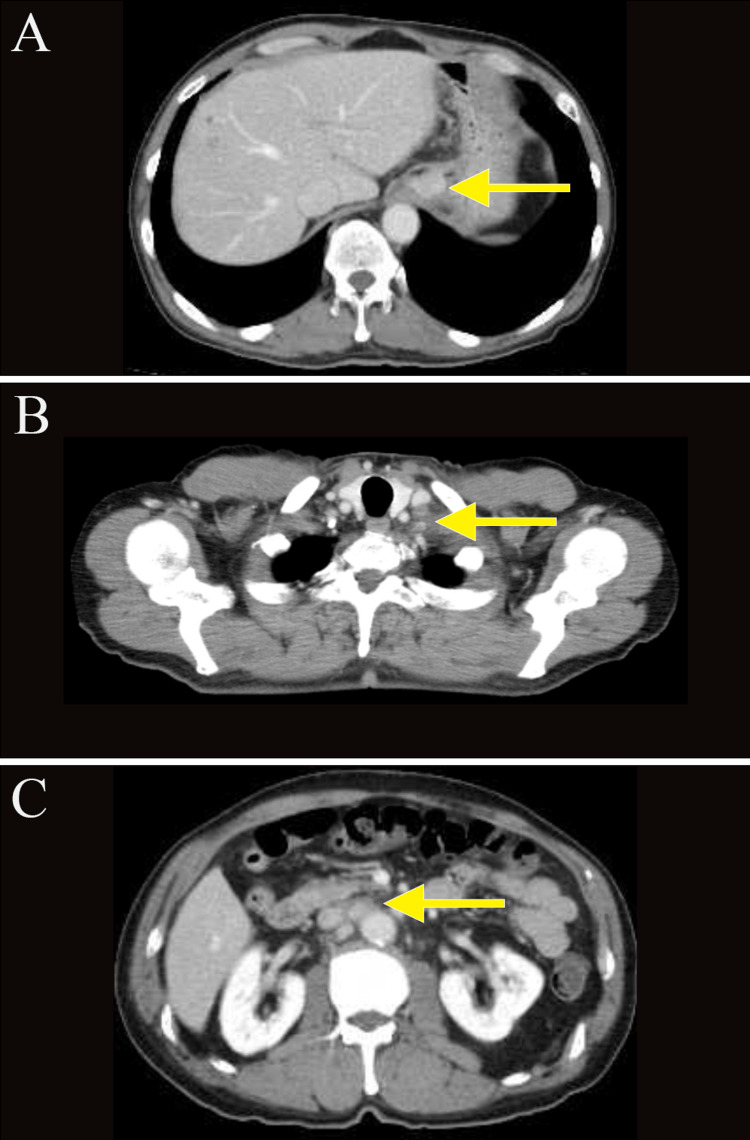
Computed tomography (A-C) Images taken before chemotherapy. Abdominal and cervical computed tomography (CT) scans revealed wall thickening at the hilum and multiple enlarged lymph nodes in the left supraclavicular fossa and around the aorta, from the hilum to the upper abdomen.
